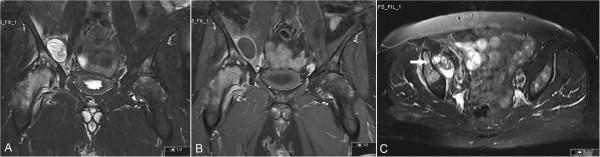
Coronal (A and B) and axial (C) magnetic resonance imaging scans. Coronal T2-weighted fat-saturated (A) and T1-weighted fat-saturated contrast-enhanced (B) magnetic resonance imaging scans showing a cystic lesion located on the medial side of the right iliac bone. The cyst is associated with the adjacent acetabulum (white arrow) on the axial fat-saturated magnetic resonance imaging scan (C).
